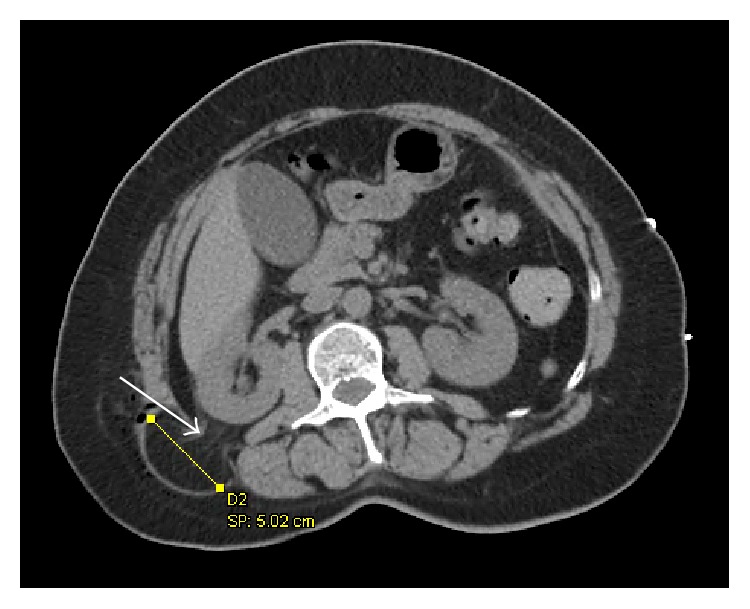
Noncontrast CT of patient's abdomen showing a right sided Grynfeltt-Lesshaft hernia. Arrow: adipose tissue herniating through defect at the upper lumbar triangle. Line with square endpoints: hernia sac diameter measurement.
